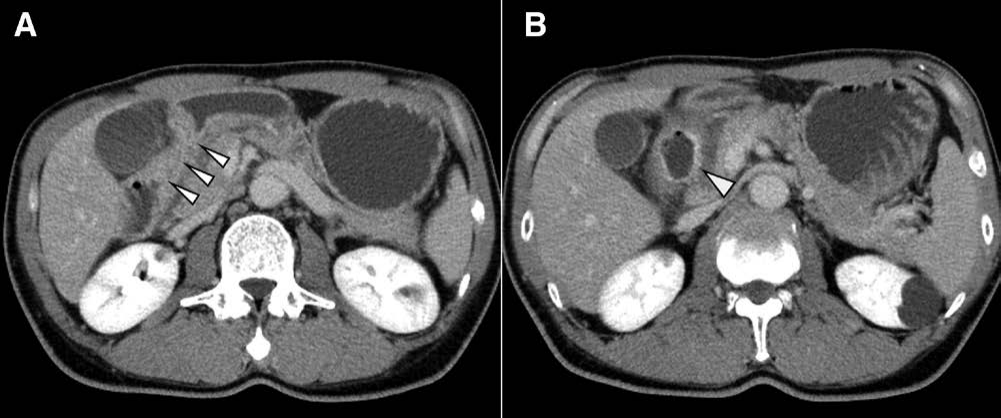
Contrast-enhanced computed tomography showed (A) wall thickening and luminal narrowing in the descending part of the duodenum (arrowheads) and (B) a cystic lesion approximately 27 × 19 mm contiguous to the duodenum (arrowhead).
